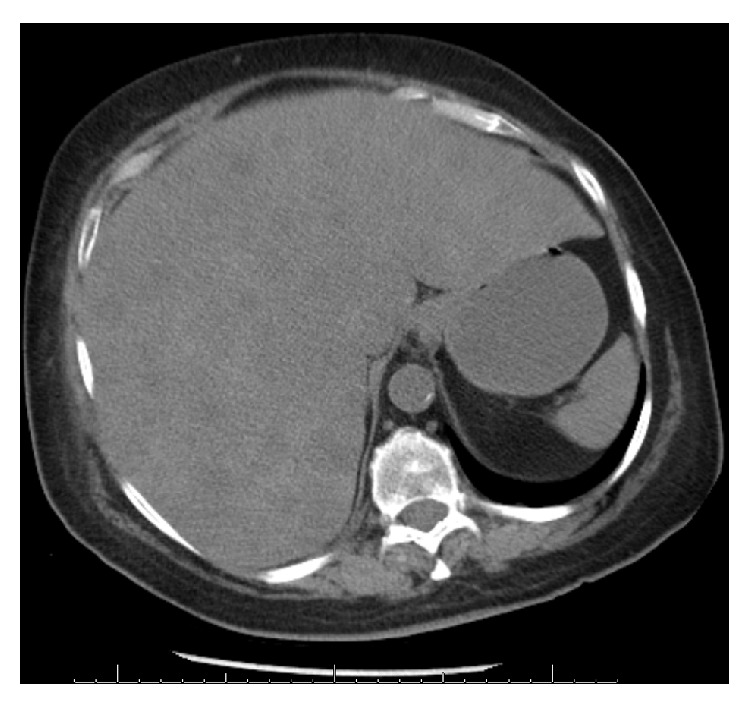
CT of the abdomen without contrast showed markedly enlarged abnormal heterogeneous liver.
